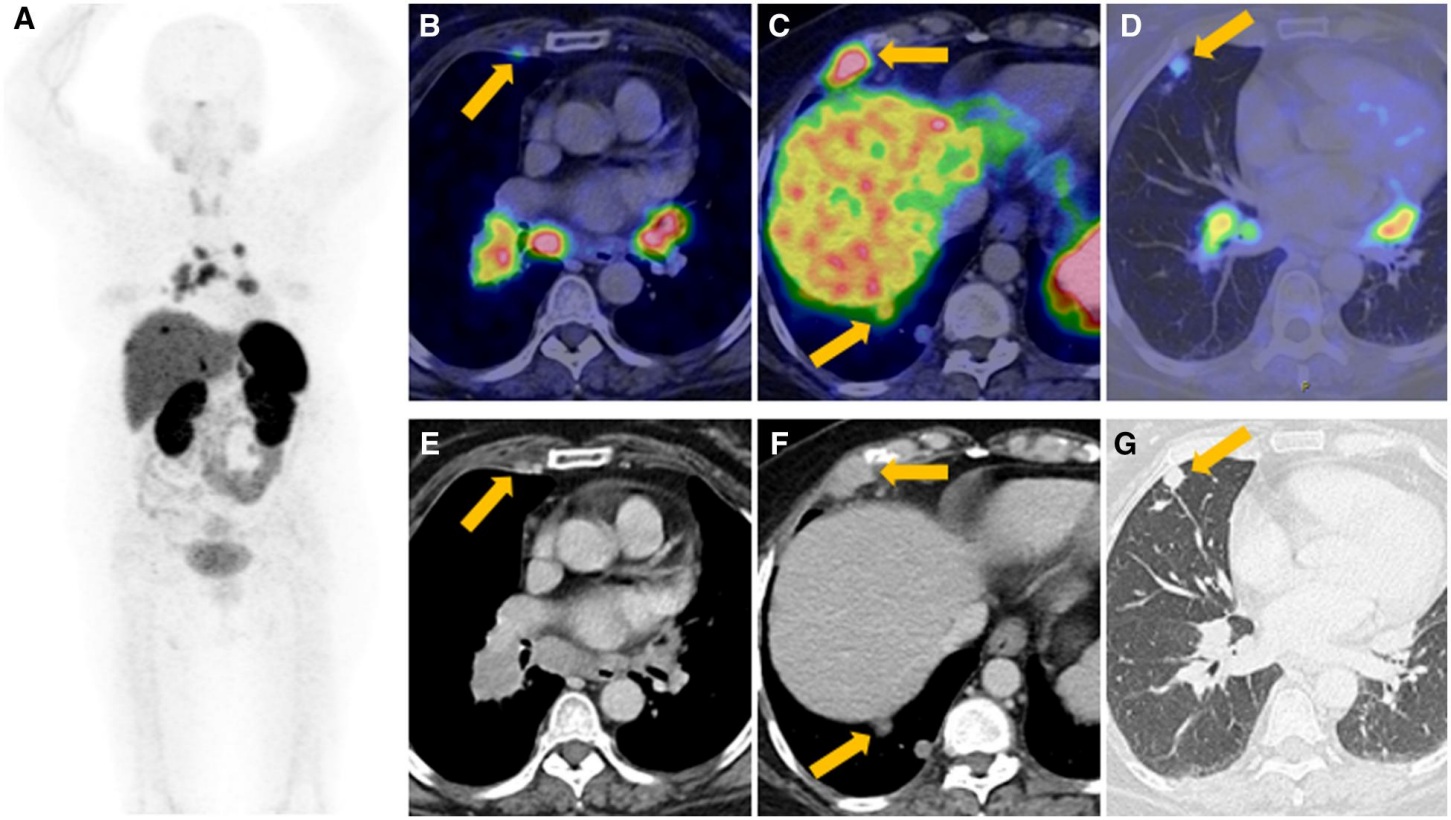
^68^Gallium DOTA-[Tyr3] octreotate PET CT ((A) maximum intensity projection; (B-D) axial hybrid; (E-G) axial CT) shows (B, E) multiple DOTATATE-avid mediastinal, bilateral interlobar and internal mammary (arrow) nodal metastases; (C, F) superior diaphragmatic and intercostal (upper arrow) nodal metastases; as well as pulmonary (arrow, D, G) and pleural (lower arrow, C, F) metastases.
